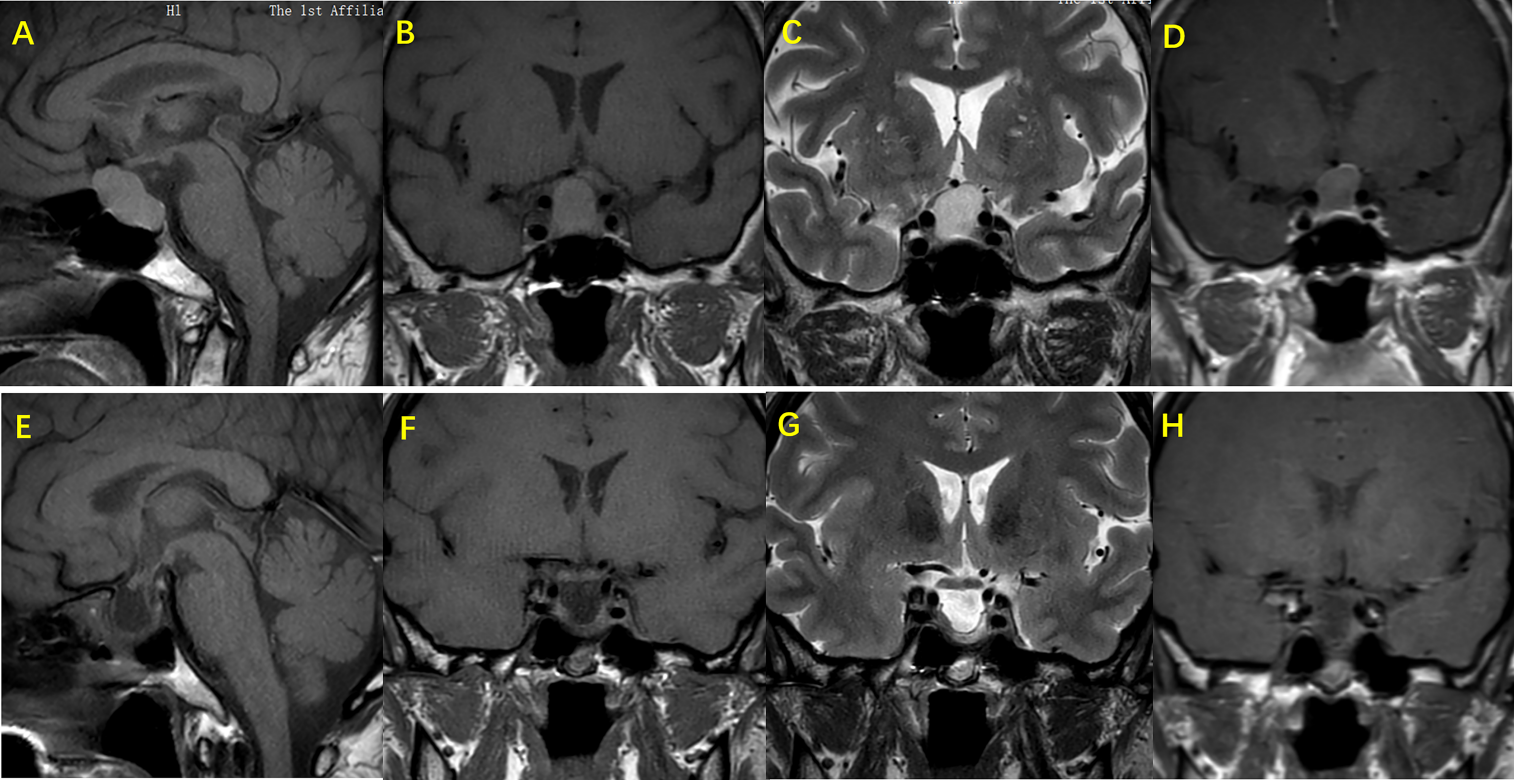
Images of MRI and gadolinium-enhanced MRI sequencing for sella region in RCC patient. Female patient, age 47, suffers headache as the main symptoms, MRI shows a snowman appearance of the RCC, the cyst present isointense on T1W imaging. (A, B) and hypertense on T2W imaging. (C) and the wall has been partialy enhanced with gadolinium administration (D) In the preoperative picture of the patient, the RCC is compressing the sella - supraselar region causing compression of optic chiasm, while pituitary stalk is not visible as the result of compression. Pictures (E–H) show the 6 months post - operation evaluation of the pituitary gland. Hypertense on T1W (E, F) and heterogeneous intensity on T2W imaging (G) present in the sela - suprasellar region, with irregular enhancement after gadolinium administration. Also, pituitary stalk appears although it has been shifted, and the compression of optic chiasm is seen relieved.
